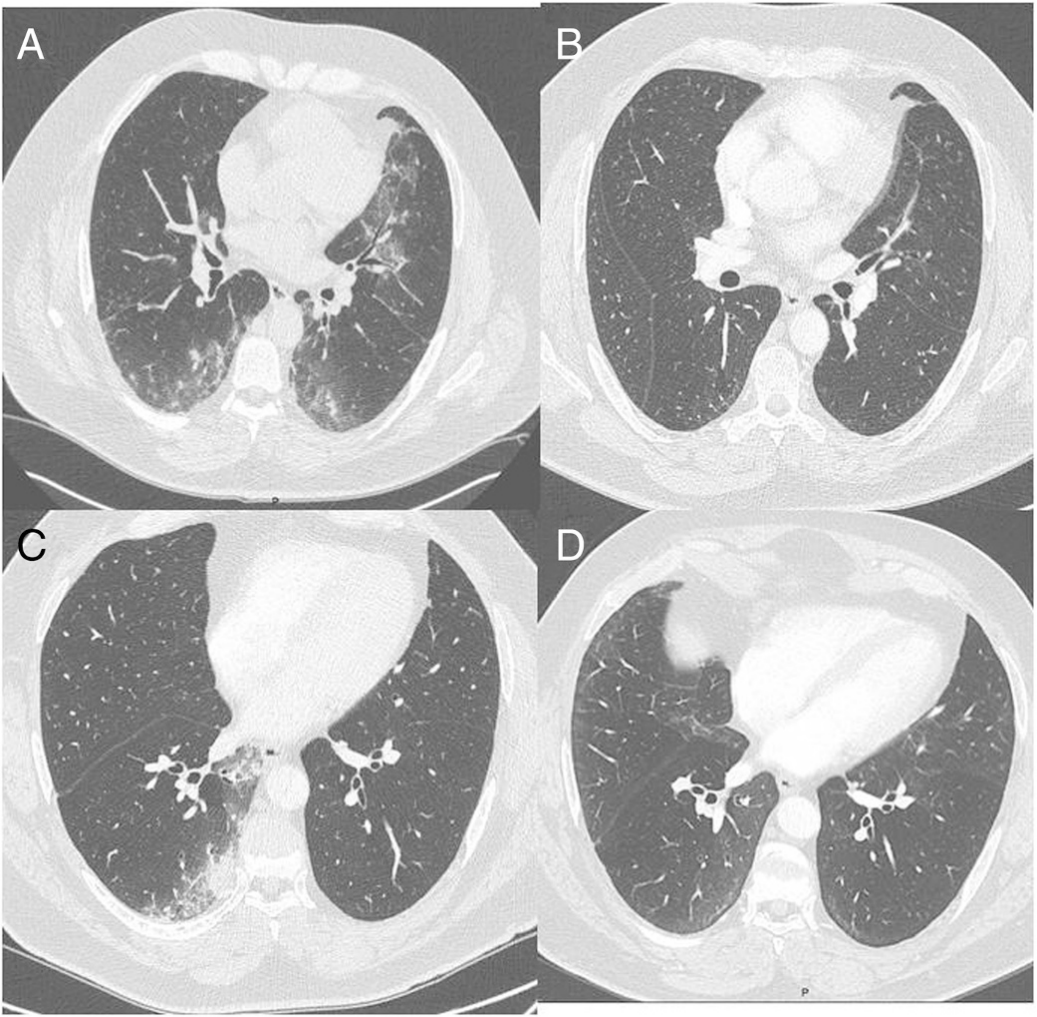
Chest CT scan (a): during the episode of acute respiratory failure after 5 months of treatment with trametinib, (b): 7 months after trametinib discontinuation and 5 months after vemurafenib initiation, (c): 15 months after vemurafenib initiation, (d): 6 months after vemurafenib discontinuation
